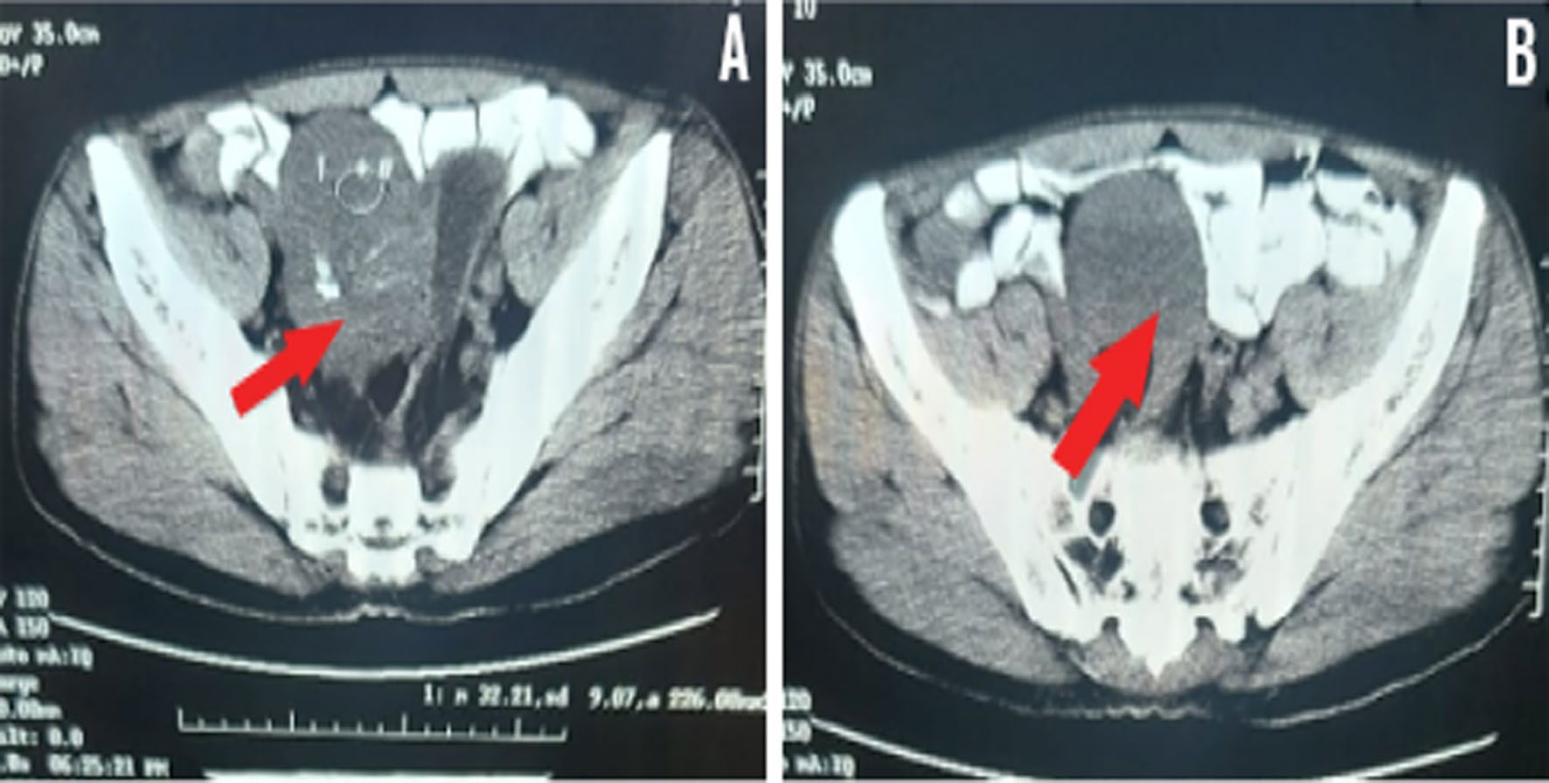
A, B Axial pelvis CT scan with intravenous injection of contrast material shows the pelvic cyst (red arrow)
